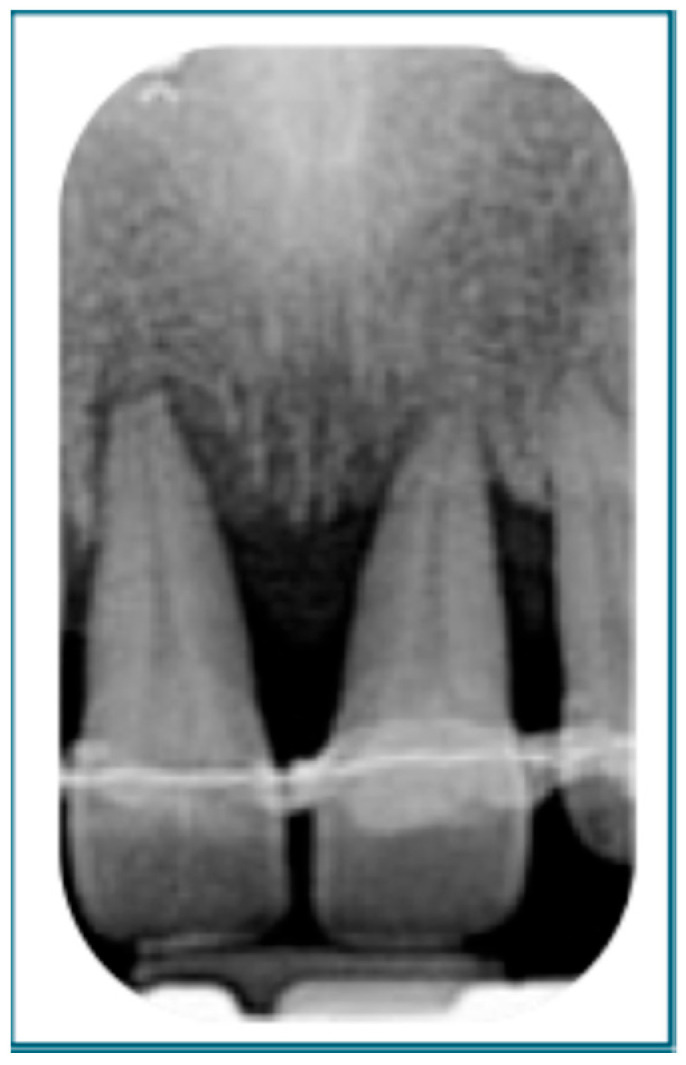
Tooth #11 with hopeless prognosis presenting radiographic bone loss approaching the root apex circumferentially.
